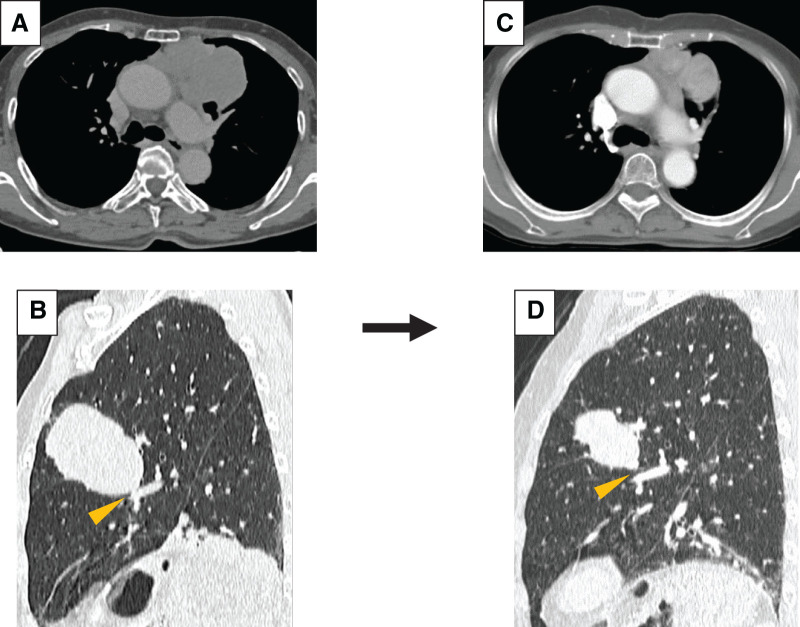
(A) Chest CT showing a 7-cm diameter mass in the anterior mediastinum. (B) Sagittal section of chest CT image. The tumor had extensive infiltration in the left upper lobe and involved A5 (arrowhead). (C) After 2 cycles of CAMP therapy, the primary tumor shrank to 6.0 cm. (D) Extent of the infiltration in the left upper lobe was reduced and A5 was free (arrowhead). CAMP, cisplatin, doxorubicin, and methylprednisolone
